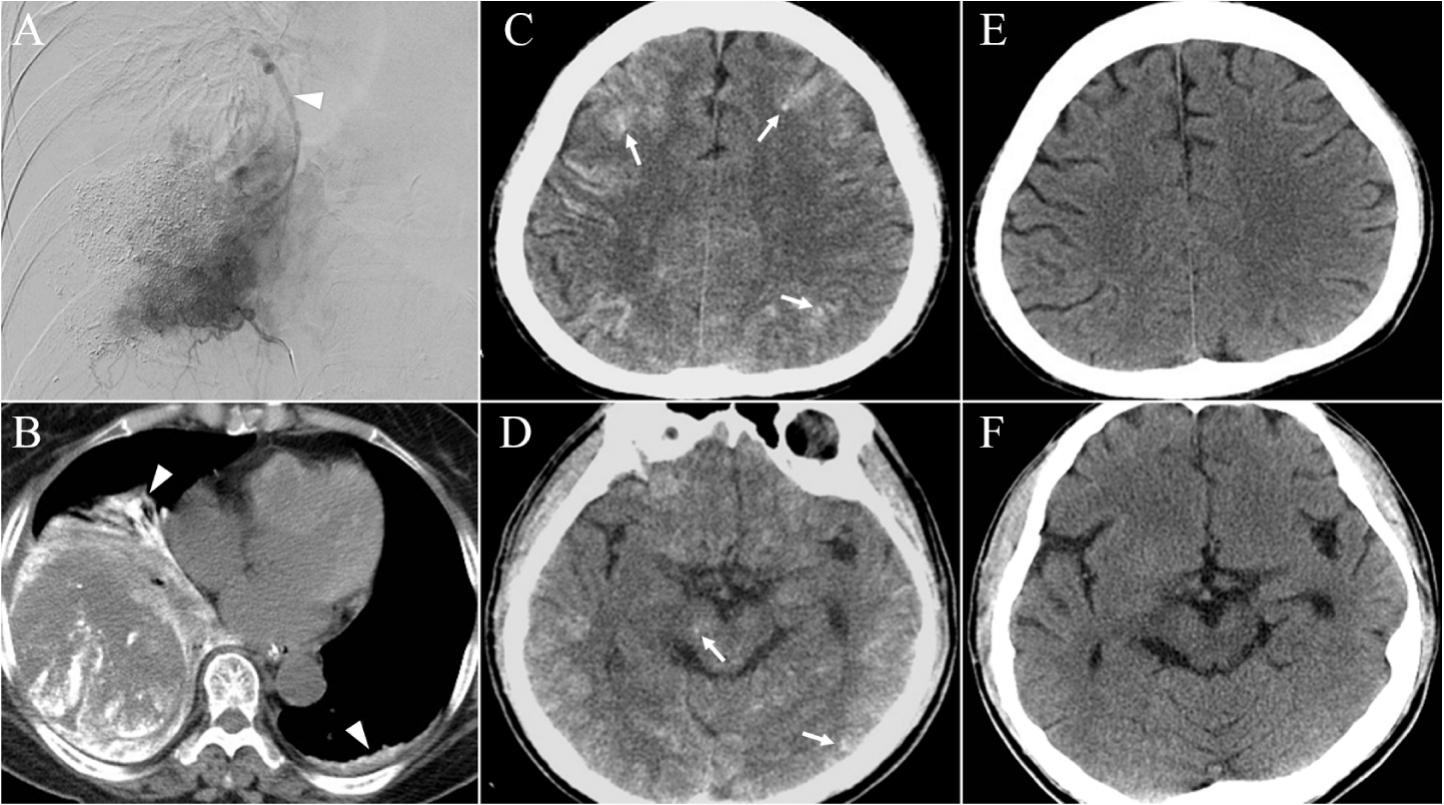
Head and abdominal imaging findings in HCC patients with pulmonary vein shunting due to direct diaphragm and pleura invasion. A right inferior phrenic artery angiogram showed prior embolized hepatic tumor with large adjacent recurrence. An early opacified pulmonary vein branch was seen (arrow head) (A); non-contrast chest CT at 2 days after CLE showed lipiodol pneumonitis at bilateral collapsed basal lung (arrow head) (B); on head non-contrast CT there were several hyperdense spots (arrow) in addition to typical disseminated lesions of increased attenuation of CLE at the cerebral hemispheres (C) and brain stem (D). At 3 weeks follow-up, head CT of the same patient showed disappearance of the previous hyperdense lesions (E & F).
